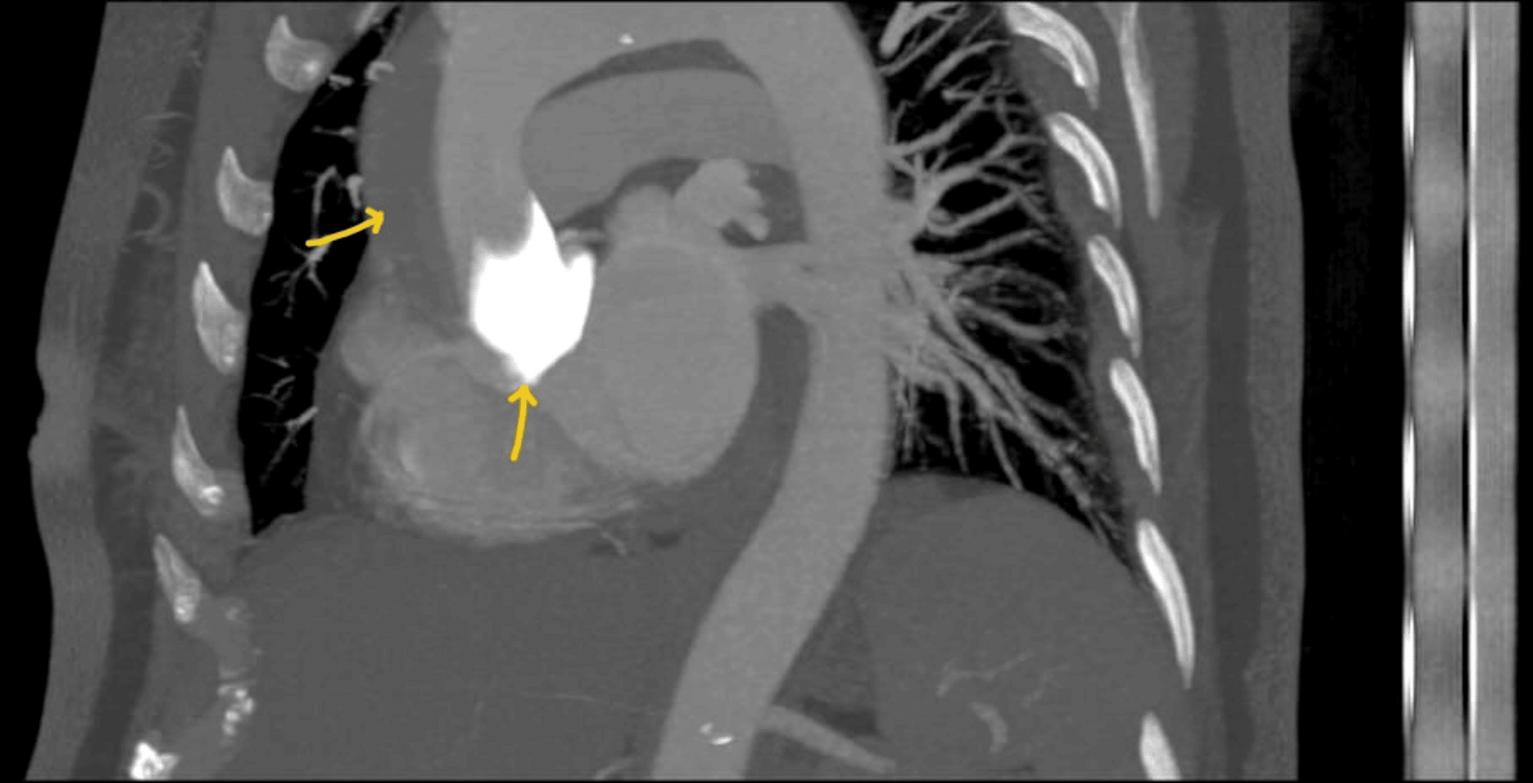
CT aortogram Arrow indicates the extravasation of contrast in dissection flap in the ascending aorta.
